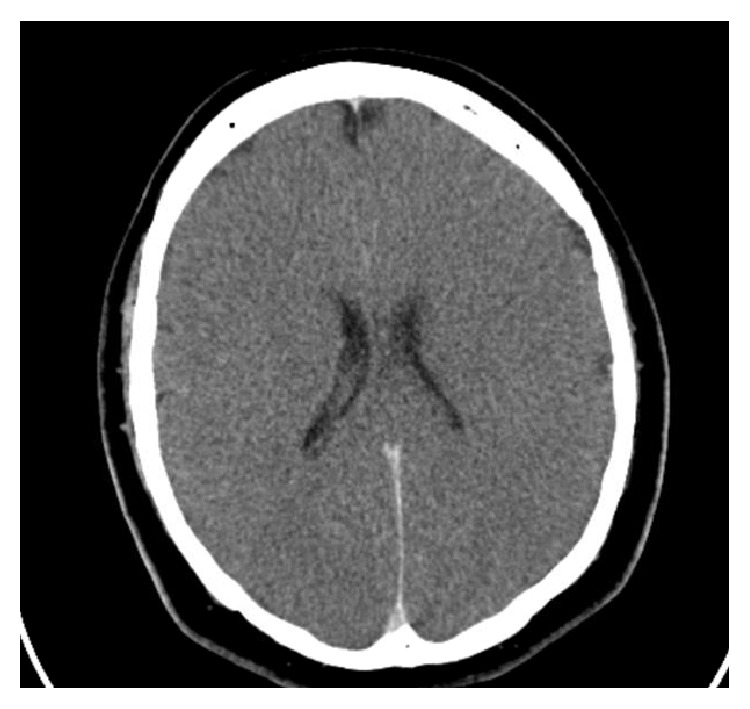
Case #1. Computed tomography of head without contrast with diffuse cerebral edema consistent with brain anoxic injury.
